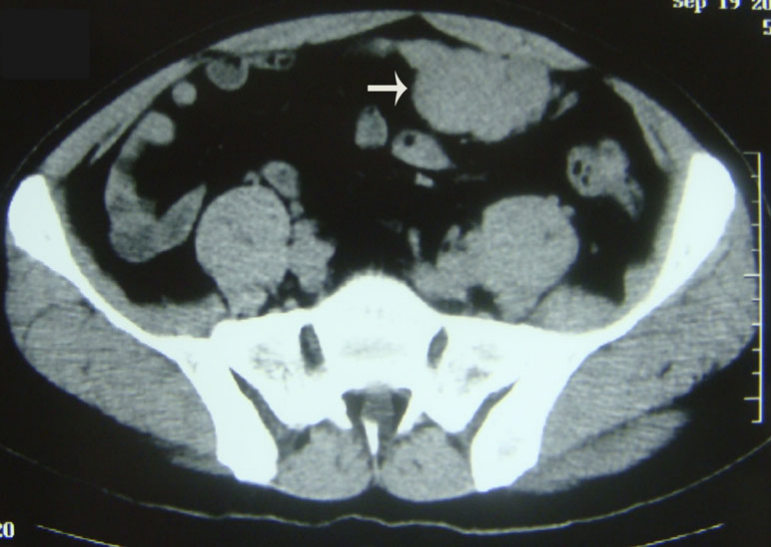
CT scan showing tumors involved the sigmoid flexure.
